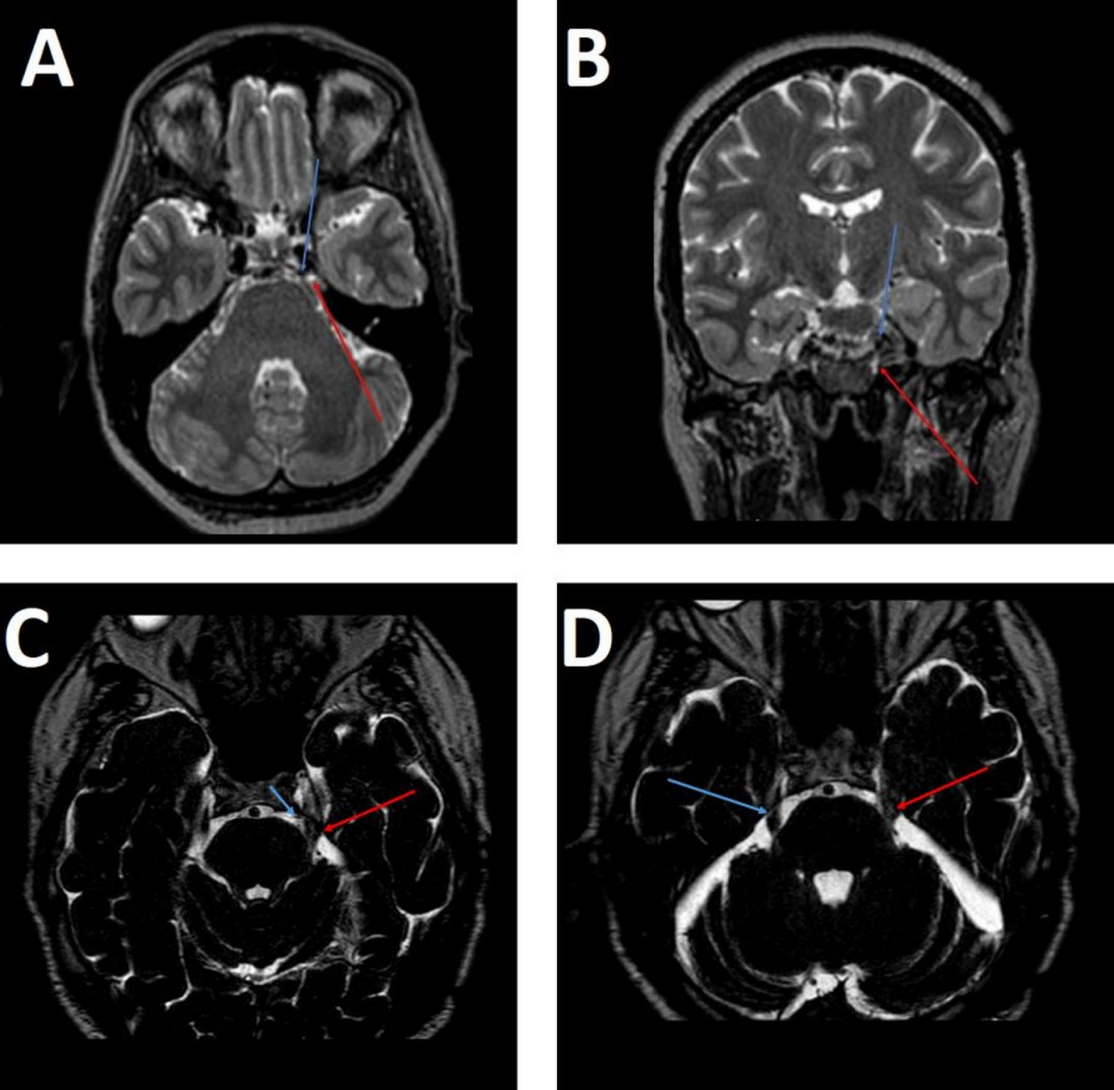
High-resolution T2-weighted magnetic resonance imaging (MRI).
